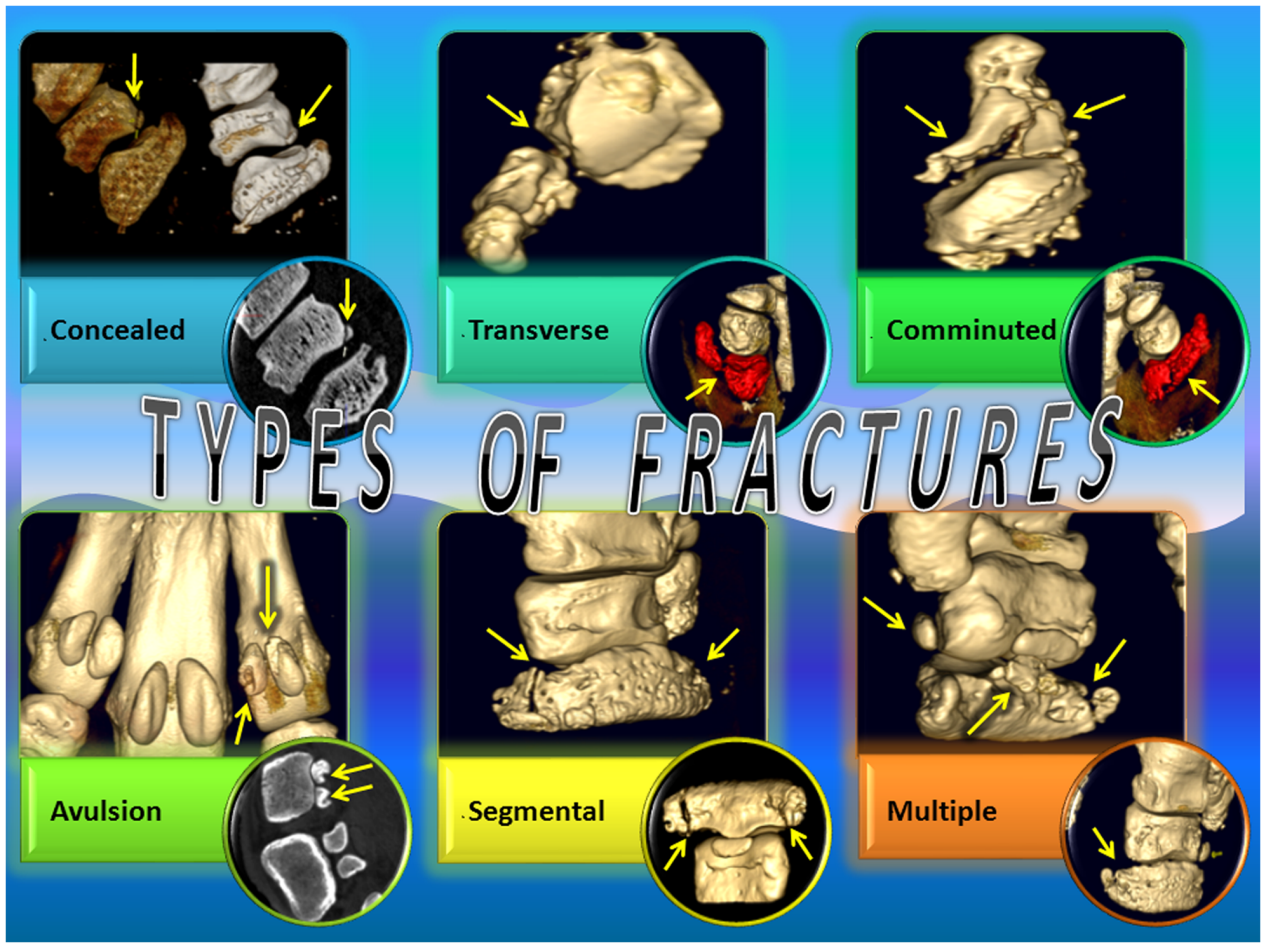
Types of fractures. Computed tomographic images of different types of fractures: *concealed fractures*, characterized by new bone production overlying the fracture line, concealing it; *transverse fractures*, complete, occurring perpendicular on the long axis of the bone; *comminuted fractures*, with minimum three bone fragments and connected fracture lines; *avulsion fractures*, separation of small fragments of bone due to traction by soft tissue attachment; *segmental fractures*, many separate fracture lines in a single bone; *multiple fractures*, fractures affecting different bones.
